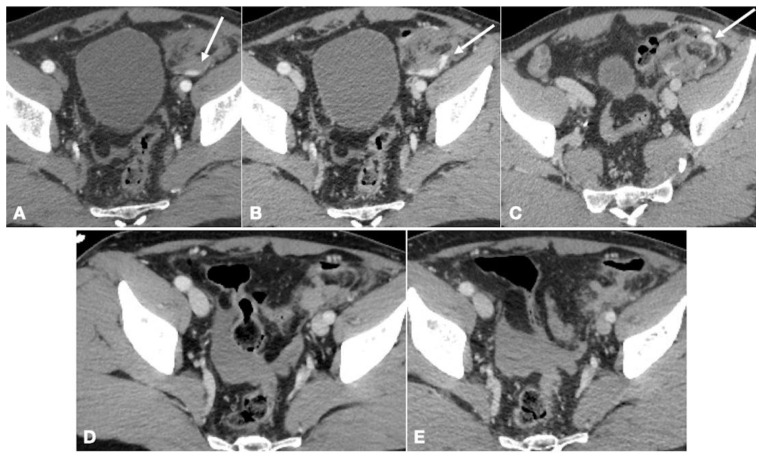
Enhanced-CT of a 54-year old male involved in major trauma (car accident). The multiphasic CT study allowed characterization of bleeding as arterial in origin, as seen on the arterial phase (A, arrow). Contrast extravasation persisted in the subsequent two phases (B,C, arrows). The patient then underwent angiography and embolization with absorbable material. Follow-up CT performed at 1st (D) and 4th (E) day after embolization showed no signs of bowel wall necrosis.
